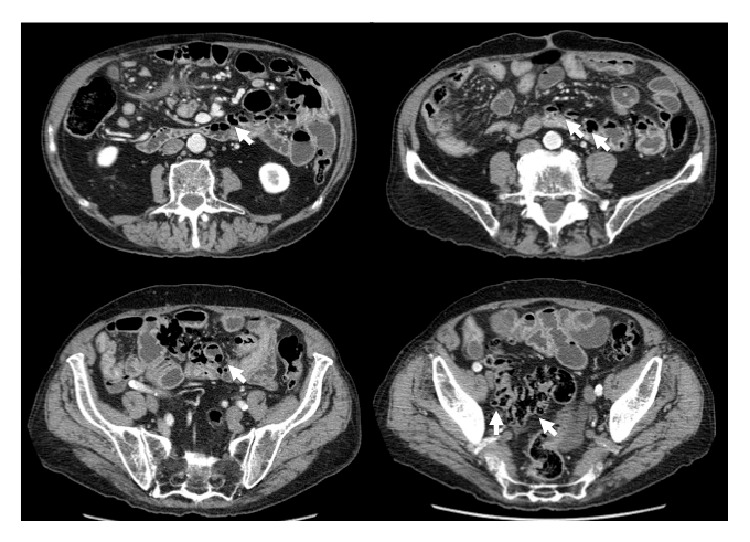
Computed tomography showing presence of gas in the intestinal wall (harrows).
